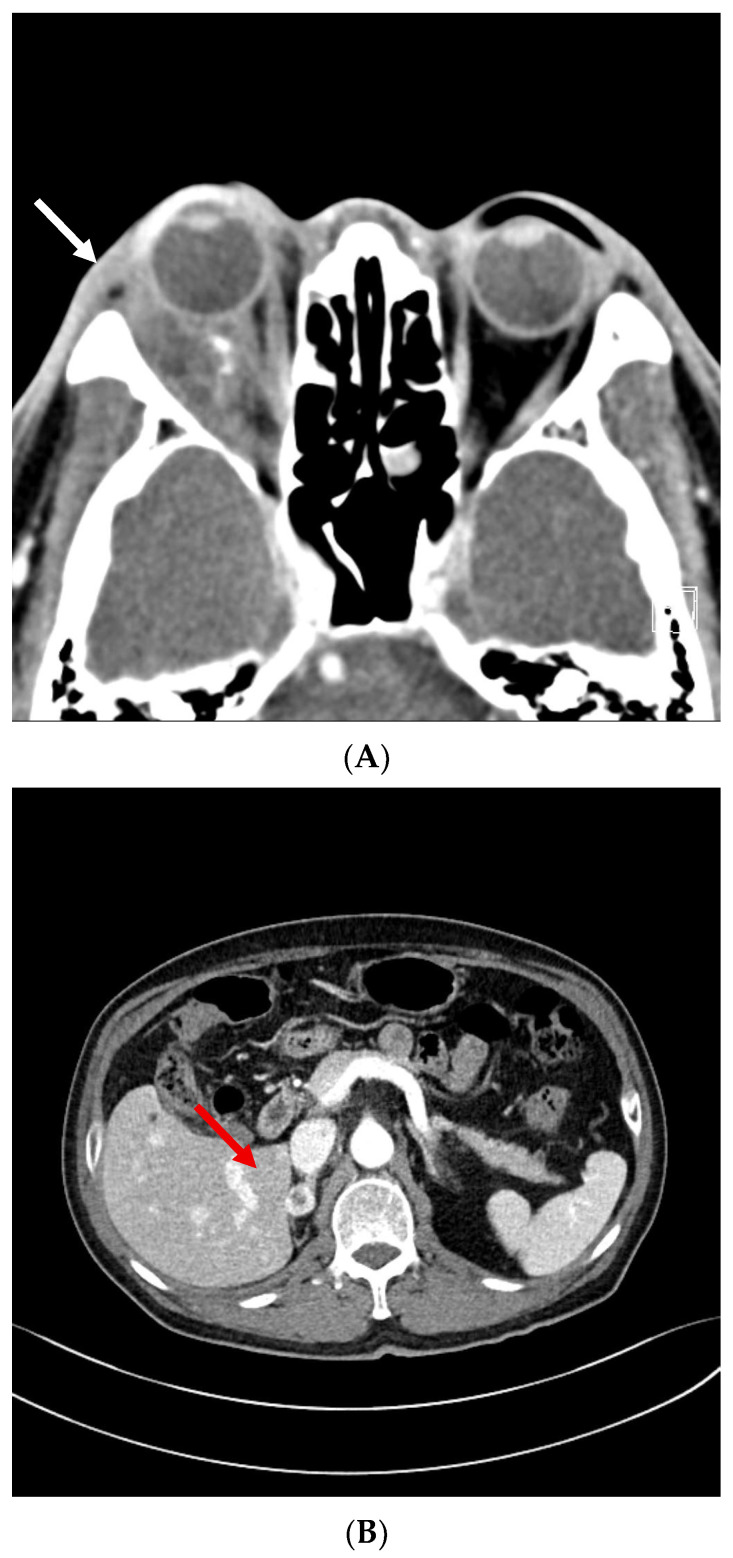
(A) Follow-up imaging demonstrated radiologic stability of the right retrobulbar mass, which had previously decreased in size on orbital CT (white arrow). (B) The right adrenal lesion also remained stable, with no progression after decreasing from 3.0 cm to 1.6 cm in maximal diameter on abdominal CT (red arrow).
